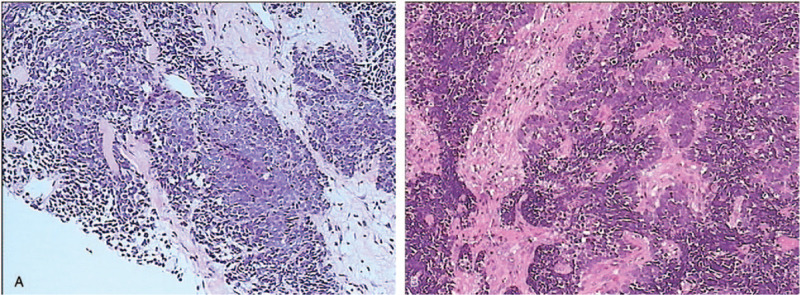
Computed tomography (CT) images of prior and postEP treatment showed therapeutic response. (A) Case 1. (B) Case 2.
